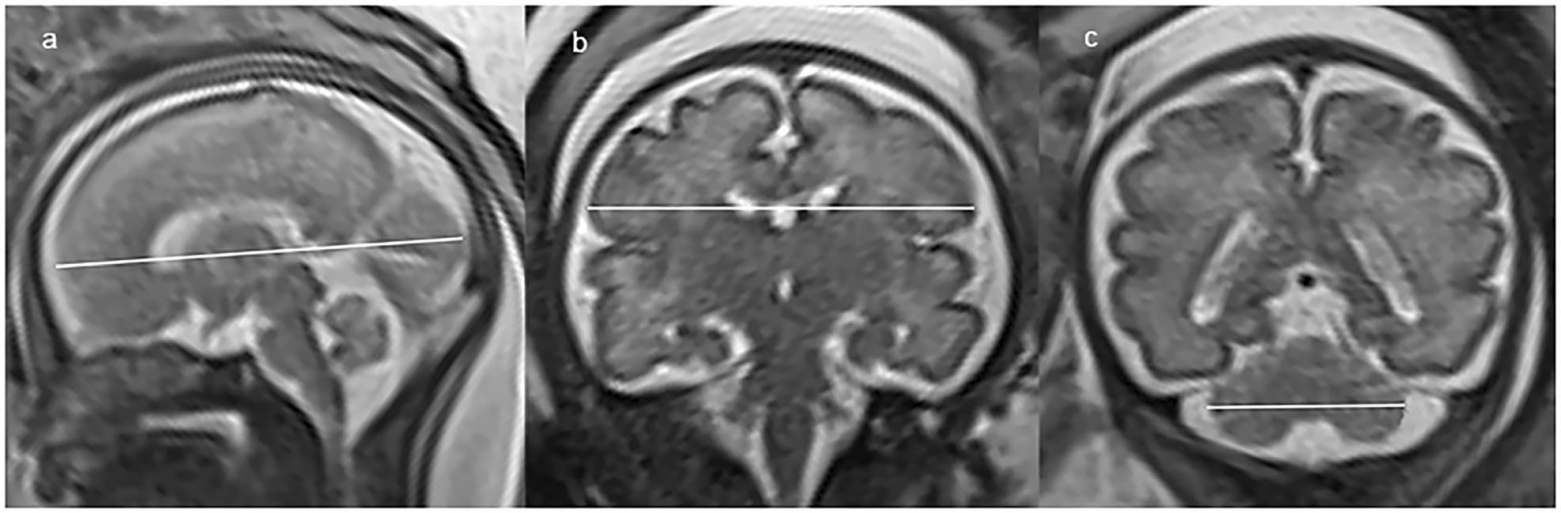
Sagittal and coronal T2-weighted imaging showing the measurements of FOD (a), BPD (b) and TCD (c) of brain itself in our study.
